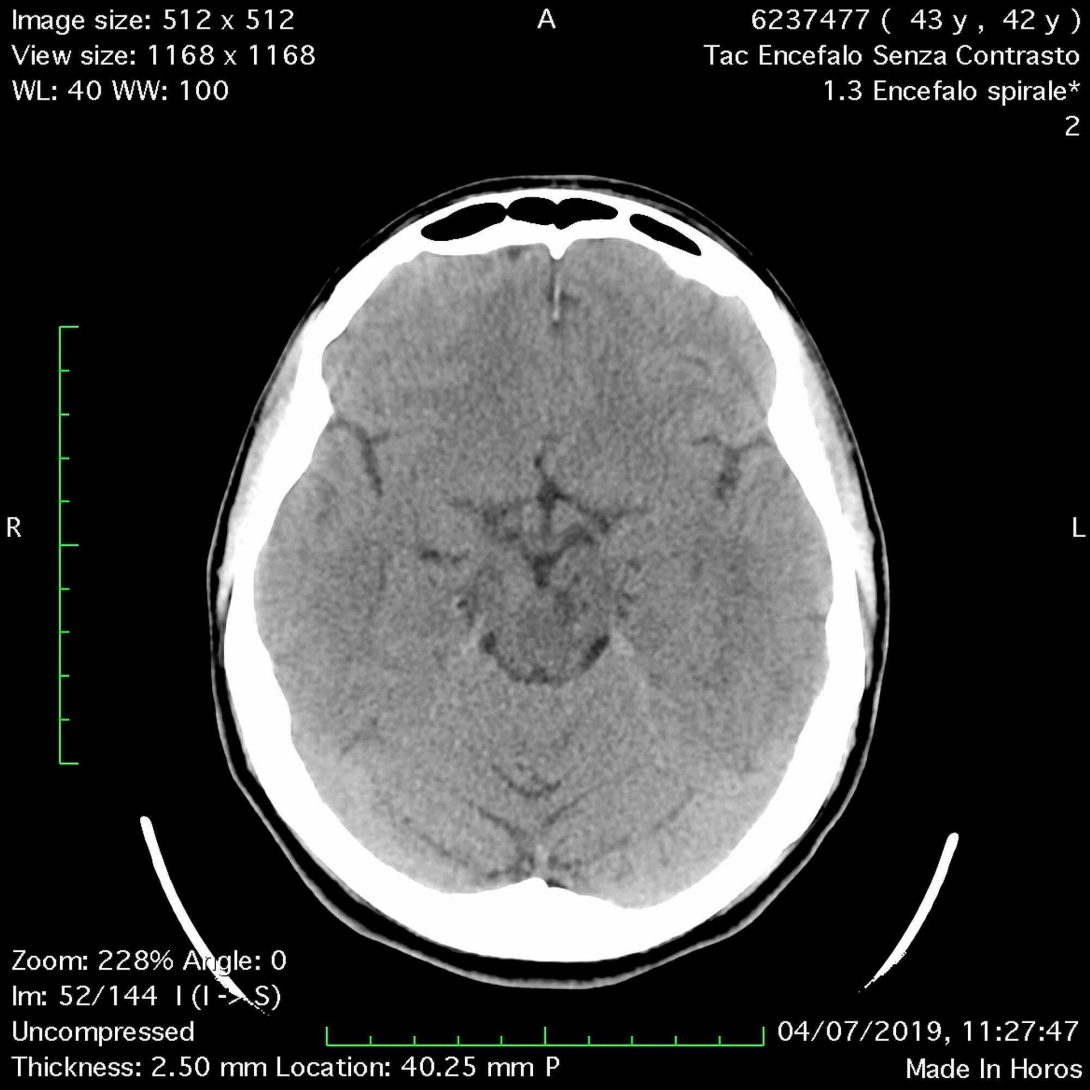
Head CT scan “after obe month”: the cystic lesion was reduced, with a punctiform residual hyperdensity.
